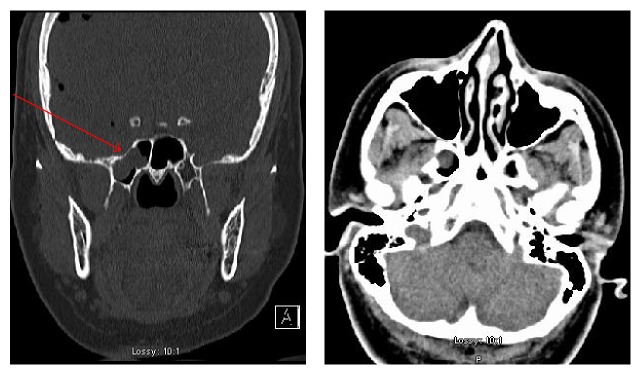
Axial and coronal Computed Tomography images of the facial bones showing right sphenoid sinus bony defect and CSF collection.
